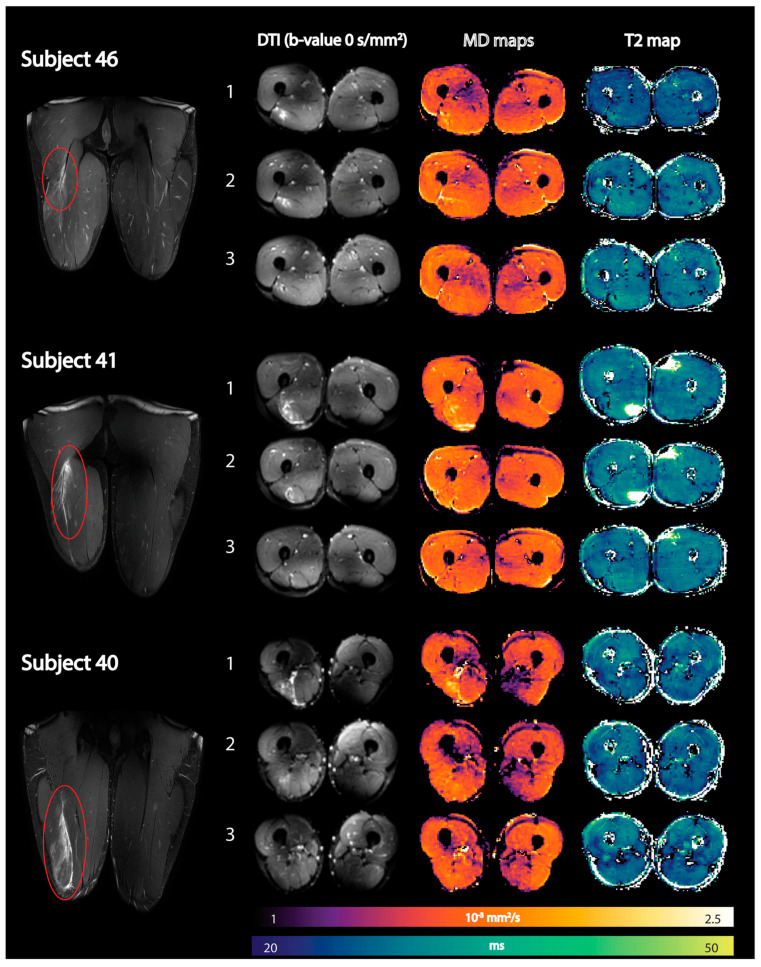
Representative images of three athletes showing coronal fat-suppressed T2-weighted images of the hamstring injury depicted by the red oval (first column) together with axial spin-echo EPI images (*b*-value = 0 s/mm^2^) (second column), reconstructed mean diffusivity (MD) maps (third column), and reconstructed qT2 maps (fourth column) at the three time points (time point 1: within 1 week postinjury; time point 2: 2 weeks after visit 1; and time point 3: at clinical return to play). DTI, diffusion tensor imaging; qT2, quantitative T2. Reproduced with permission from the authors in Ref. [130].
